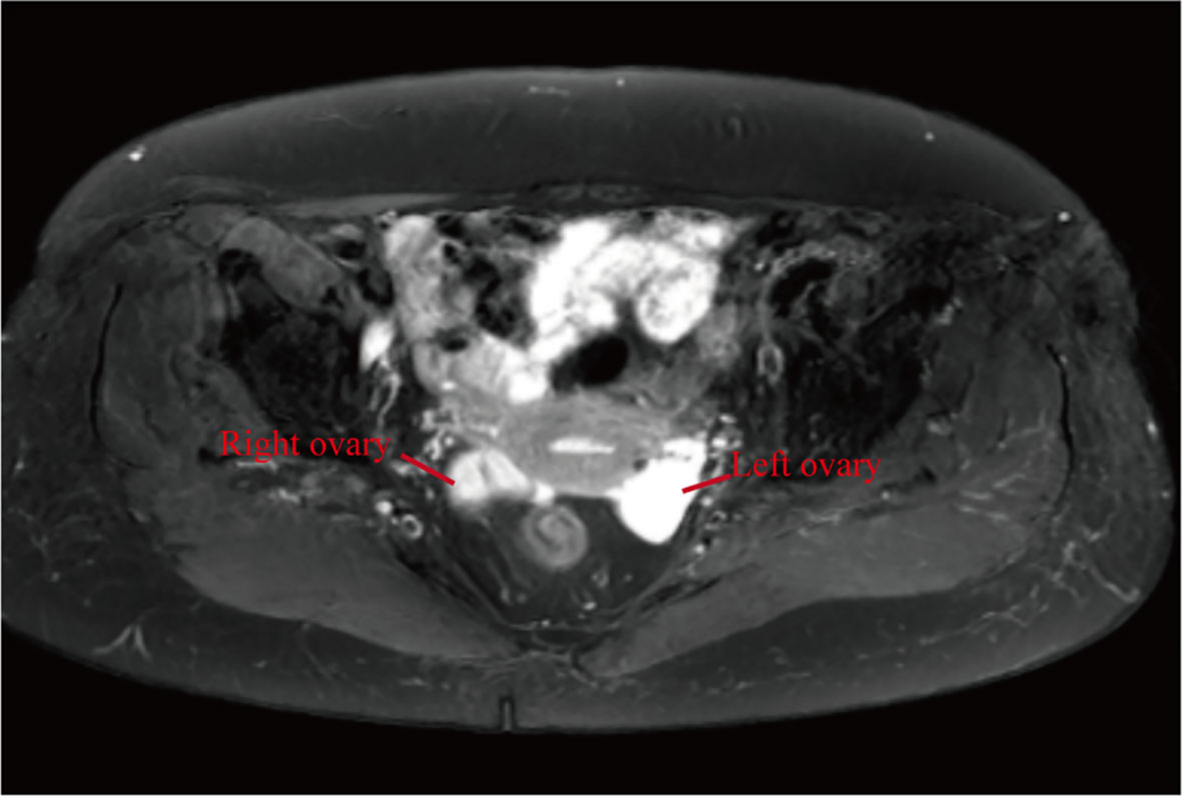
MRI pelvis plain scan and enhancement. Bilateral adnexal area cystic abnormal signal.
